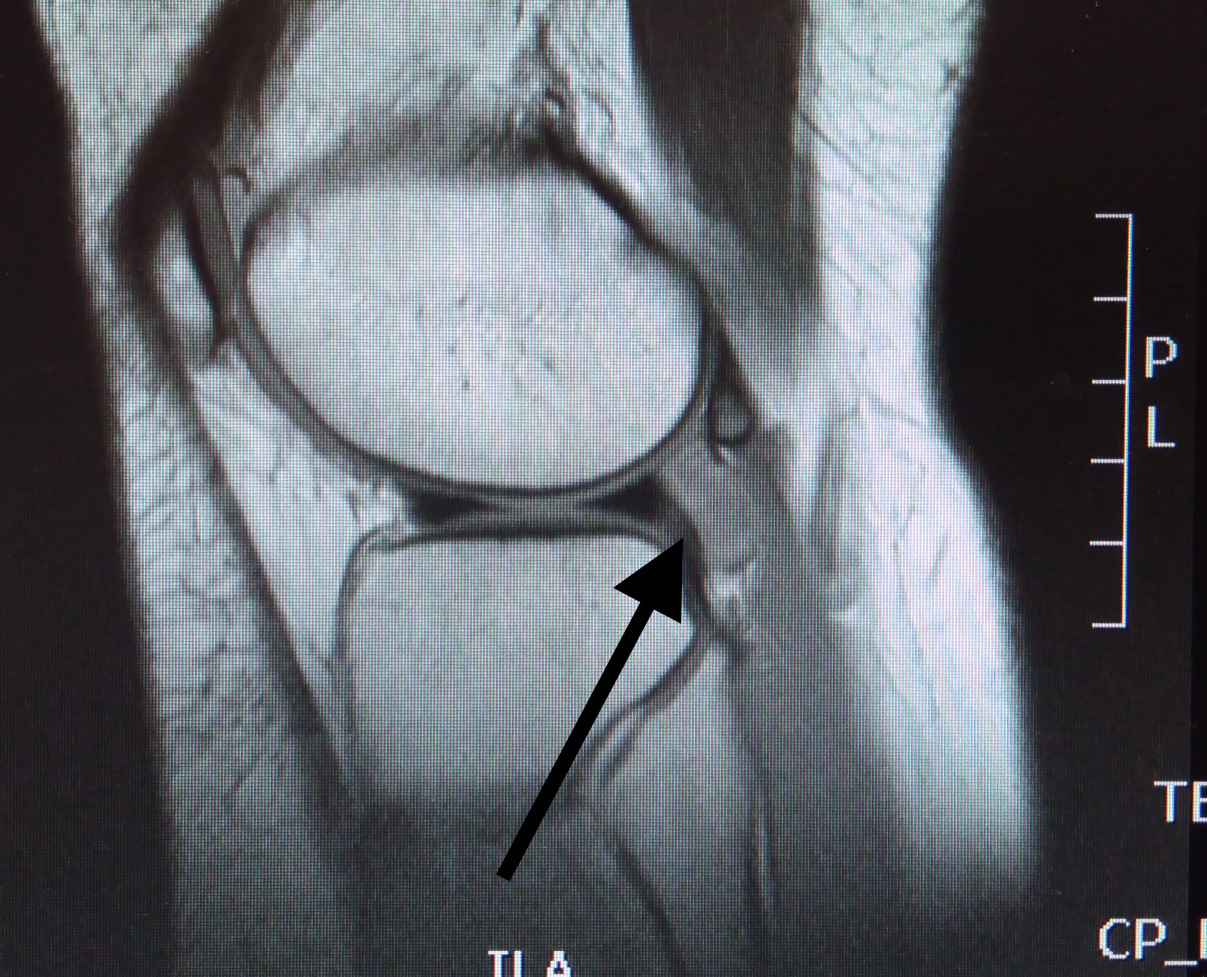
MRI of giant cell tumour MRI (T1 weighted) sequence: 2.6 cm in craniocaudal length, 2 cm in the transverse plane. MRI: Magnetic resonance imaging.
